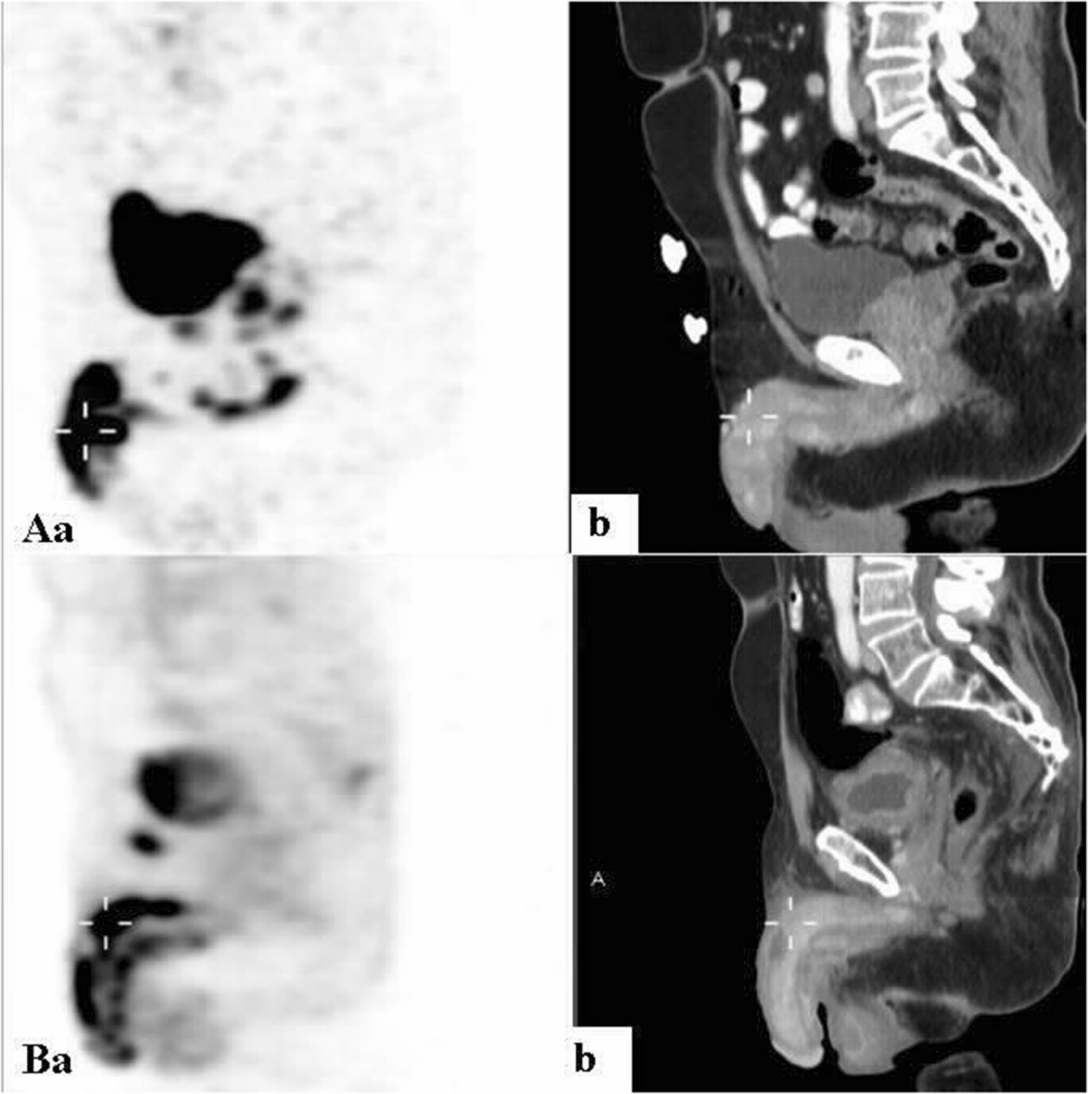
Diffuse non-homogeneous increased penile uptake on PET (a) with corresponding soft tissue lesions infiltrating the penis on CT (b) sagittal slices of: (A) A Ga-68 PSMA scan of a 68-year-old man with metastatic adenocarcinoma of the prostate, who had undergone robot assisted laparoscopic radical prostatectomy, with a Gleason’s score of 8 and PSA of 4.3 (patient #3, Table 1). (B) An 18F-FDG scan of a 72-year-old man with metastatic adenocarcinoma of the colorectum (patient #17, Table 1).
